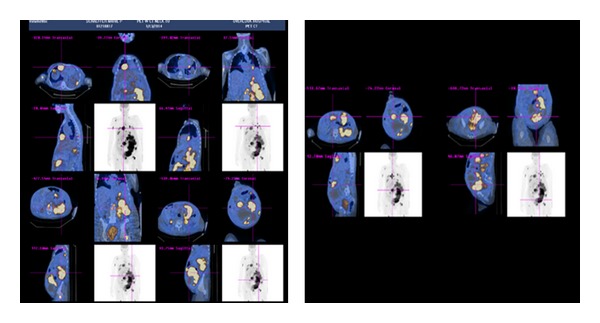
WHOLE BODY PET-CT showing multiple areas of hypermetabolic activity in the abdomen with hypermetabolic bony lesions suspicious of extensive metastatic disease.
